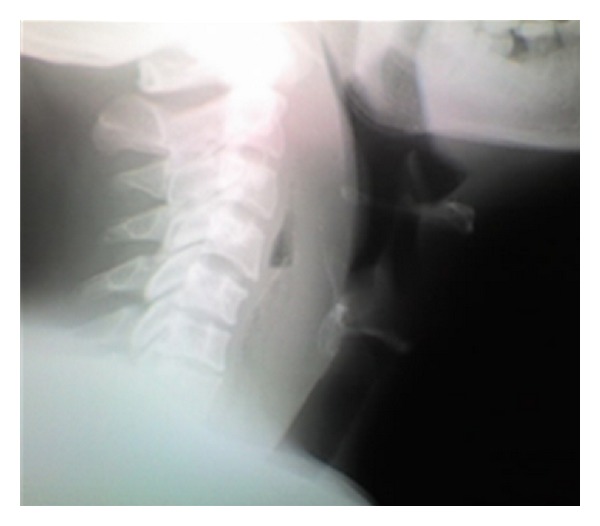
Lateral neck radiographic image of a patient who developed retropharyngeal abscess secondary to fish bone ingestion trauma.
